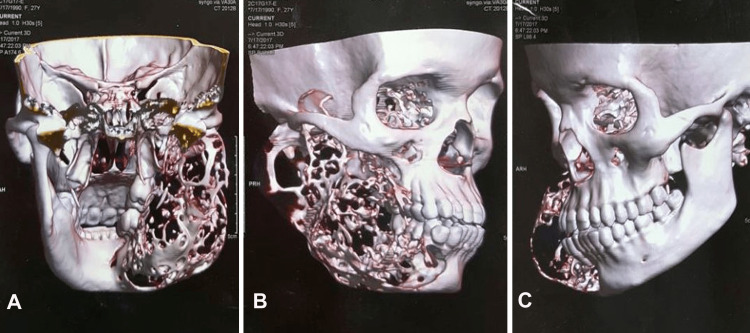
A CT scan showing infiltrative borders of the lesion A: Posterior view; B: Right side view; C: Left side view
